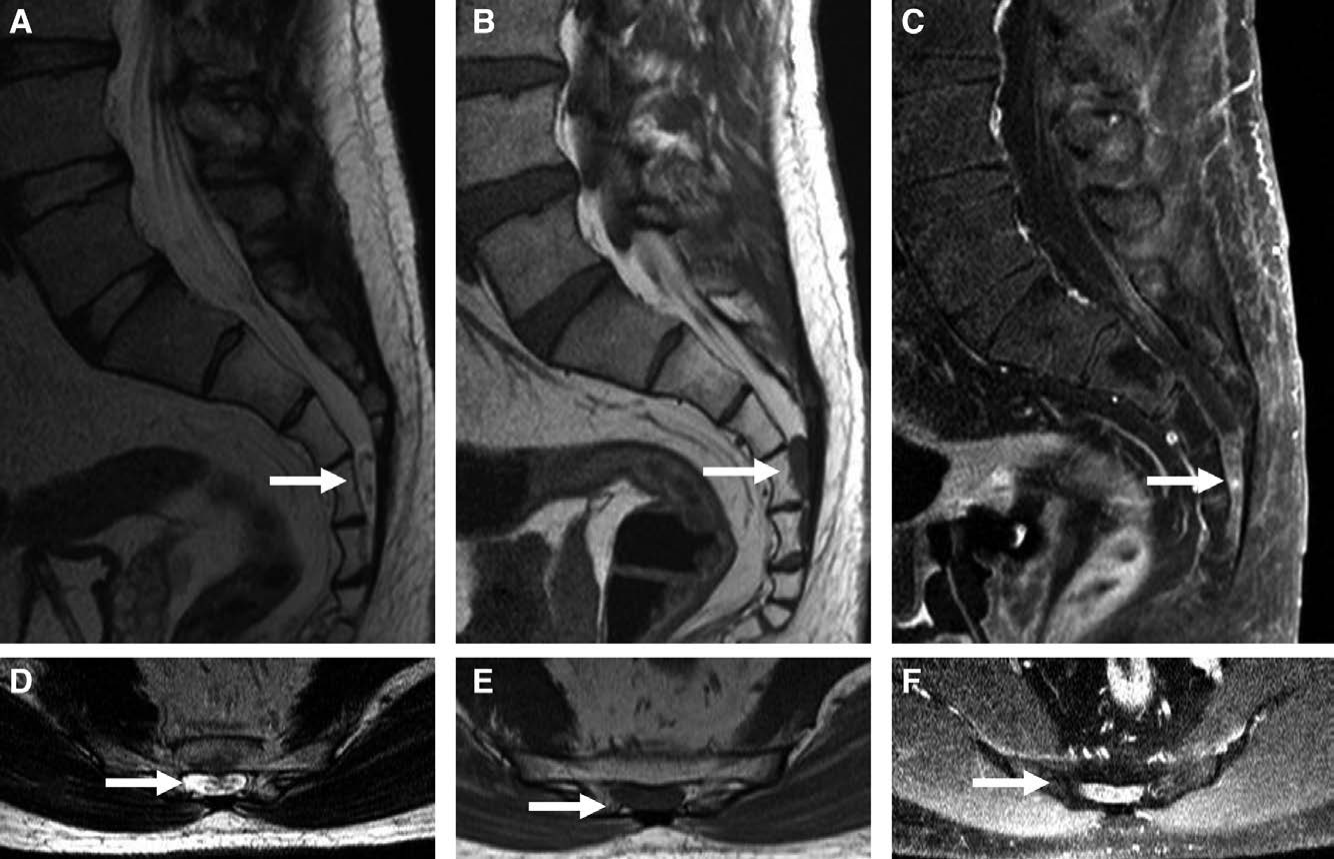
Sagittal and Axial T2-weighted, T1-weighted, and T1-weighted contrast-enhanced images. Hyperintense T2-weighted and isointense T1-weighted images show a perineural cyst with acute hemorrhage that originated from the perineurium at the S4–S5 levels (A, B, D, and E).
